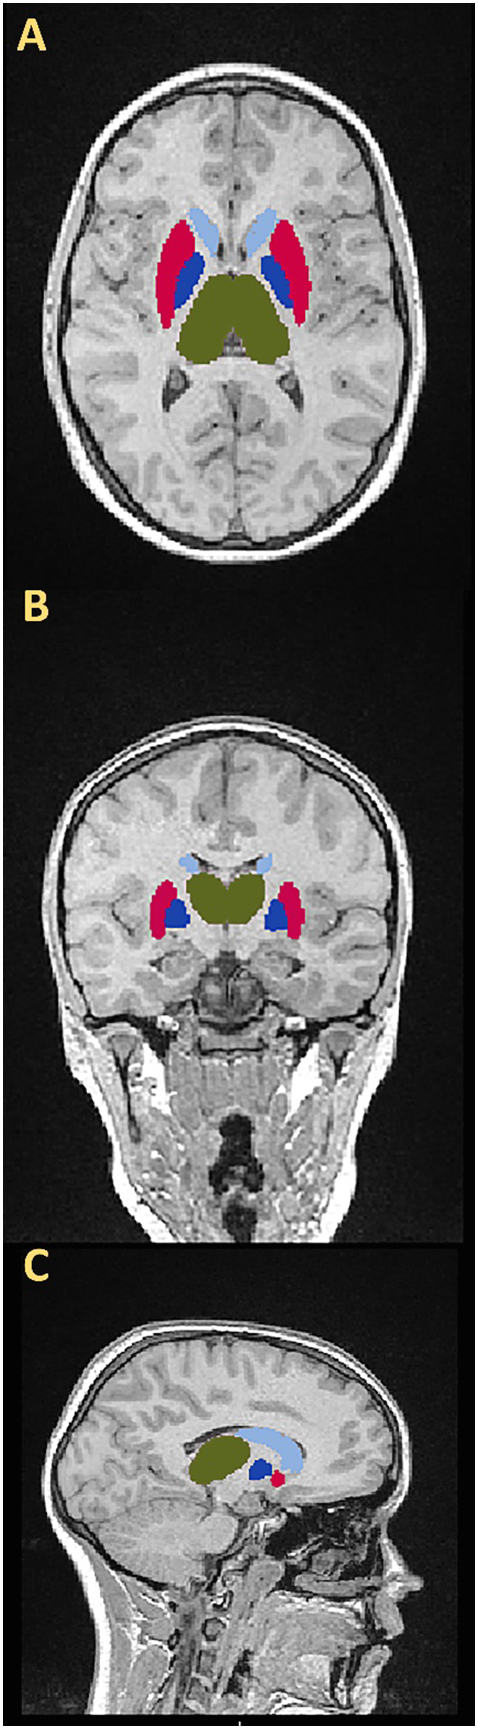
Segmented subcortical nuclei volumes of a control participant overlaid on the respective head MRI. Three planes are represented: A = axial, B = coronal, C = sagittal. Subcortical nuclei are color coded: dark green = thalami, light blue = caudate nuclei, red = putamina, dark blue = pallidi.
